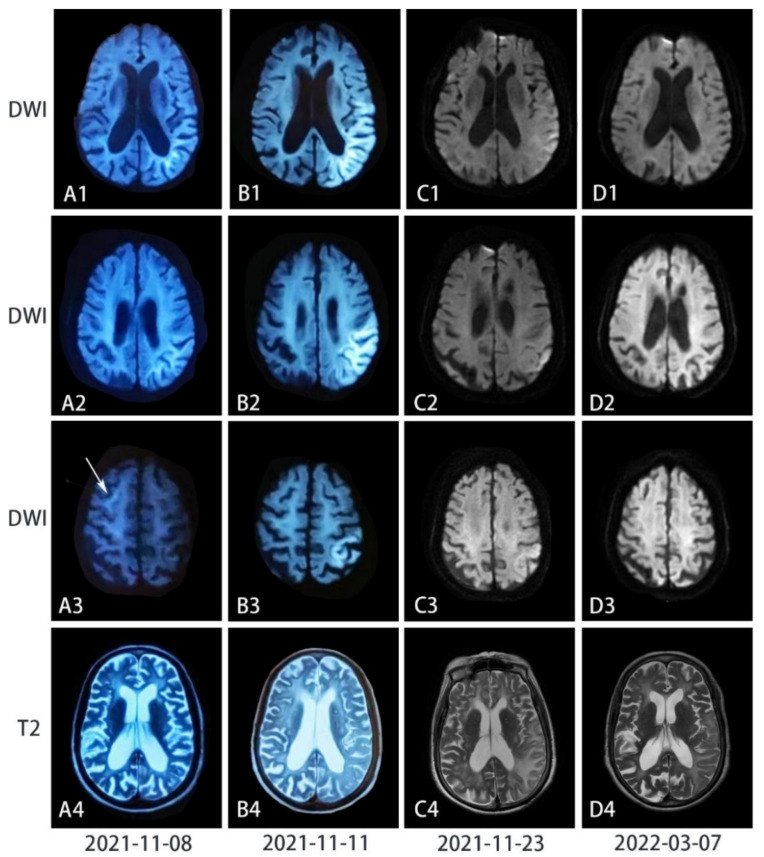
Chronological series of T2-weighted and diffusion-weighted images (DWI) images of the patient’s brain. (A) Seven days after the disease onset when she had cognitive impairment, mixed aphasia, and muscle weakness, DWI showed curvilinear periventricular hyperintensity, while the T2-weighted image showed diffuse brain atrophy. (B) Two days after her symptoms exacerbated when she could be only bedridden, curvilinear lesions had transformed into confluent high-intensity lesions on DWI along the cortex in the temporal occipitoparietal lobe (in B1), where severe encephalopathy was also observed on the T2-weighted images. However, curvilinear hyperintensity in A3 disappeared. (C) Half a month after the peak and the treatment of pulse intravenous methylprednisolone, most high-intensity signals on DWI were eliminated but leukoencephalopathy and cortical edema on the T2-weighted scan were exacerbated. (D) Four months after the symptoms onset, high-intensity signals on DWI have vanished, while hyperintensity reduced with improved brain edema on T2-weighted images.
